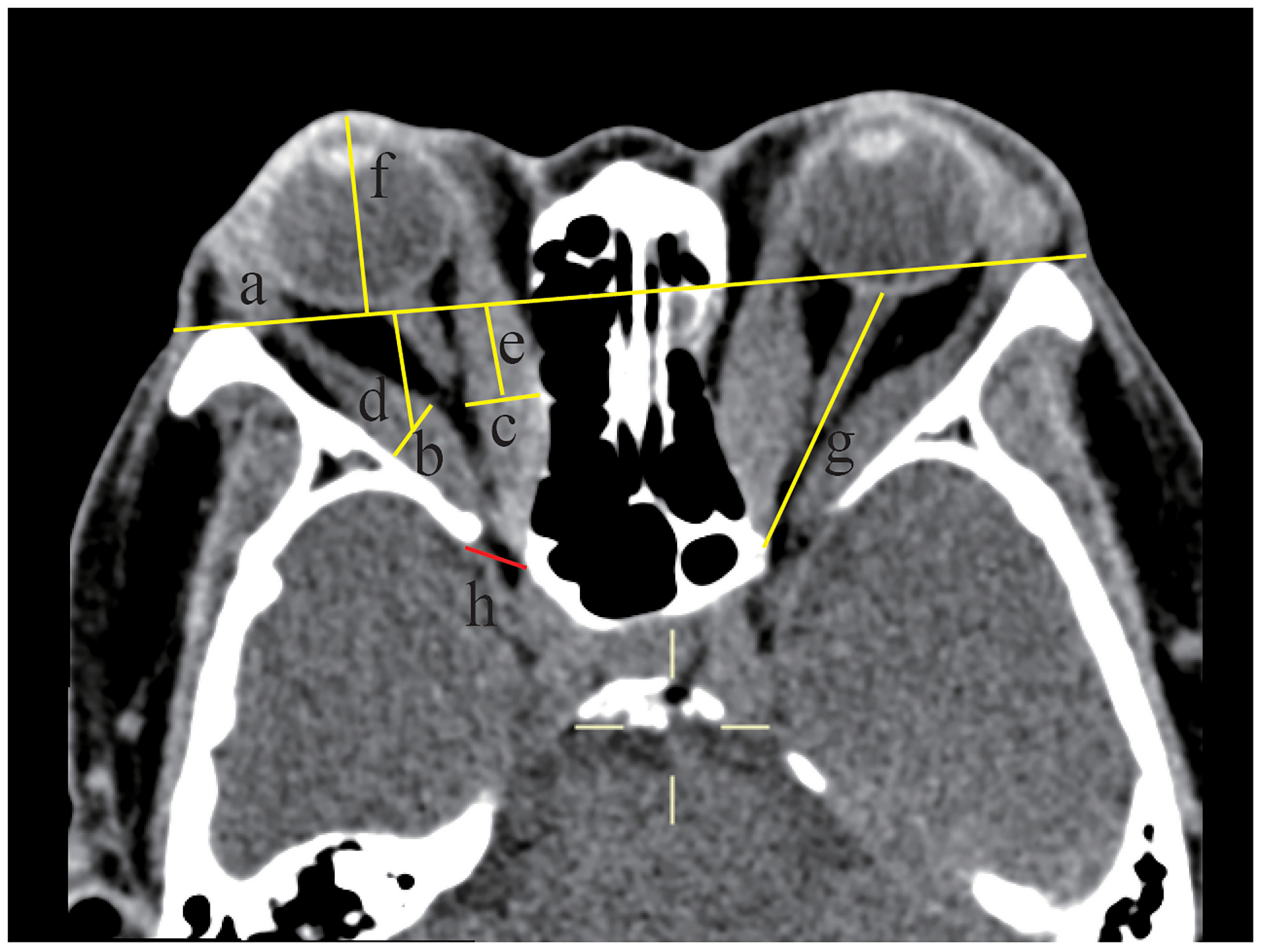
Soft tissue structures were measured by computed tomography (CT) at the axial section of a 58-year-old female dysthyroid optic neuropathy (DON) patient. The axial section at the midglobe level showed the interzygomatic line (IZ) (labeled line a) and maximum horizontal diameters of the right lateral rectus muscle (labeled line b and the length was 9.11 mm) and the medial rectus muscle (labeled line c and the length was 10.48 mm). The distance from the midpoint of the maximum muscular diameter of the lateral rectus muscle (labeled line d and the length was 17.47 mm) and medial rectus muscle (labeled line e and the length was 13.88 mm) to the IZ was recorded. Proptosis of the right eyeball was measured from the center of the anterior cornea to the IZ (labeled line f and the length was 24.66 mm). The optic nerve stretch of the left eyeball was measured from the retrobulbar optic nerve to the orbital apex point (labeled line g and the length was 36.21 mm). Intracranial fat prolapse was present in the right eye (labeled h), and the red line connected the most inner border of the sphenoid wing and the most anterior border of the sphenoid body groove.
